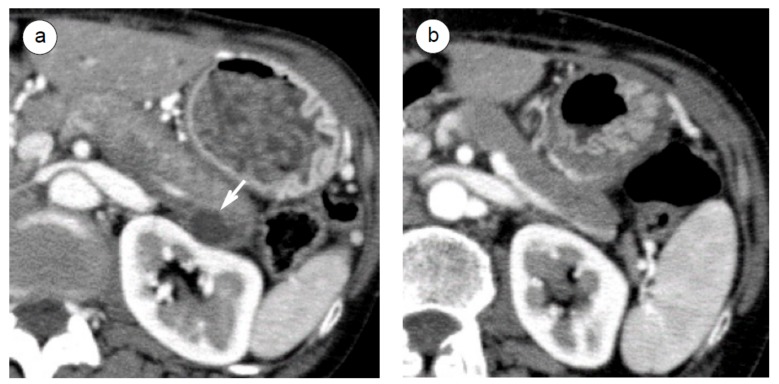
A steroid response in a pancreatic cyst of an AIP patient. Computed tomography demonstrated a unilocular cyst (arrow) at the pancreas tail, 15 mm in size, at the initial diagnosis of AIP (a). The cyst disappeared after steroid initiation (b).
